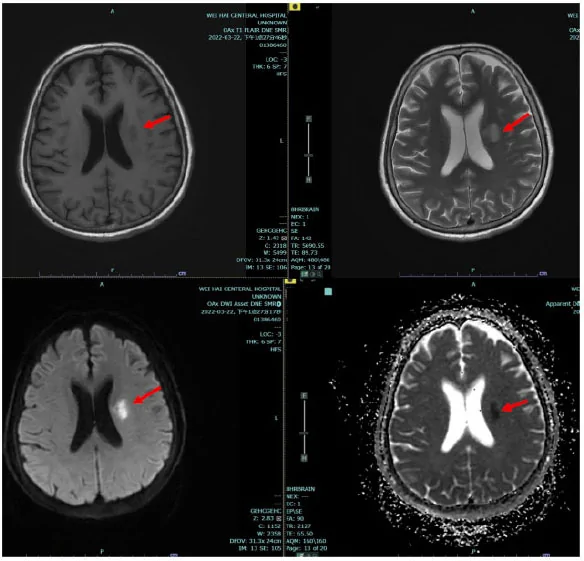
Brain MRI from March 22, 2022, shows patchy areas of prolonged T1 and T2 signal intensities in the left basal ganglia, with increased signal on DWI and low signal on ADC.
